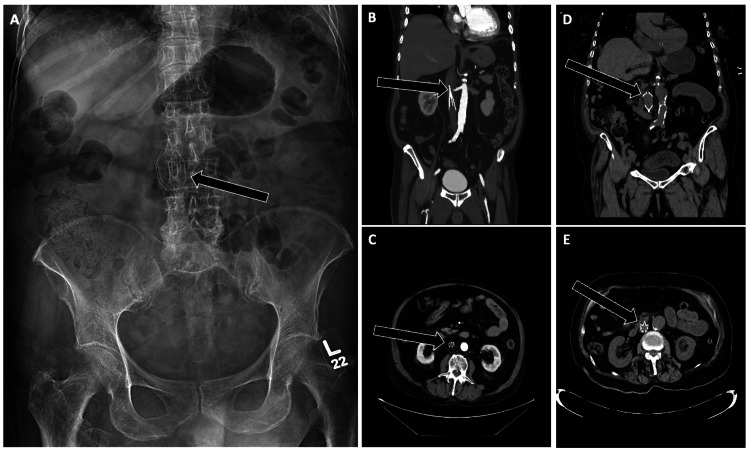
Imaging of Properly Positioned Inferior Vena Cava (IVC) Filters Imaging demonstrating appropriate positioning of various IVC filters. (A) Radiograph showing an “Optease” filter at the L3-L4 vertebral level (arrow). (B, C) Coronal and axial CT images of a “Greenfield” filter appropriately deployed within the infrarenal IVC (arrows). (D, E) Coronal and axial CT views of a “Mobin-Uddin” filter in the expected infrarenal position (arrows). Proper identification of filter type and verification of infrarenal positioning are essential to ensure effective protection against pulmonary embolism and to avoid complications such as tilt or caval penetration.
